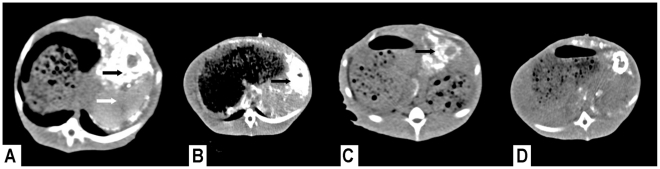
Serial CT imaging findings after TAE. Figure 3A CT scan obtained immediately after TAE. ATONs have accumulated
